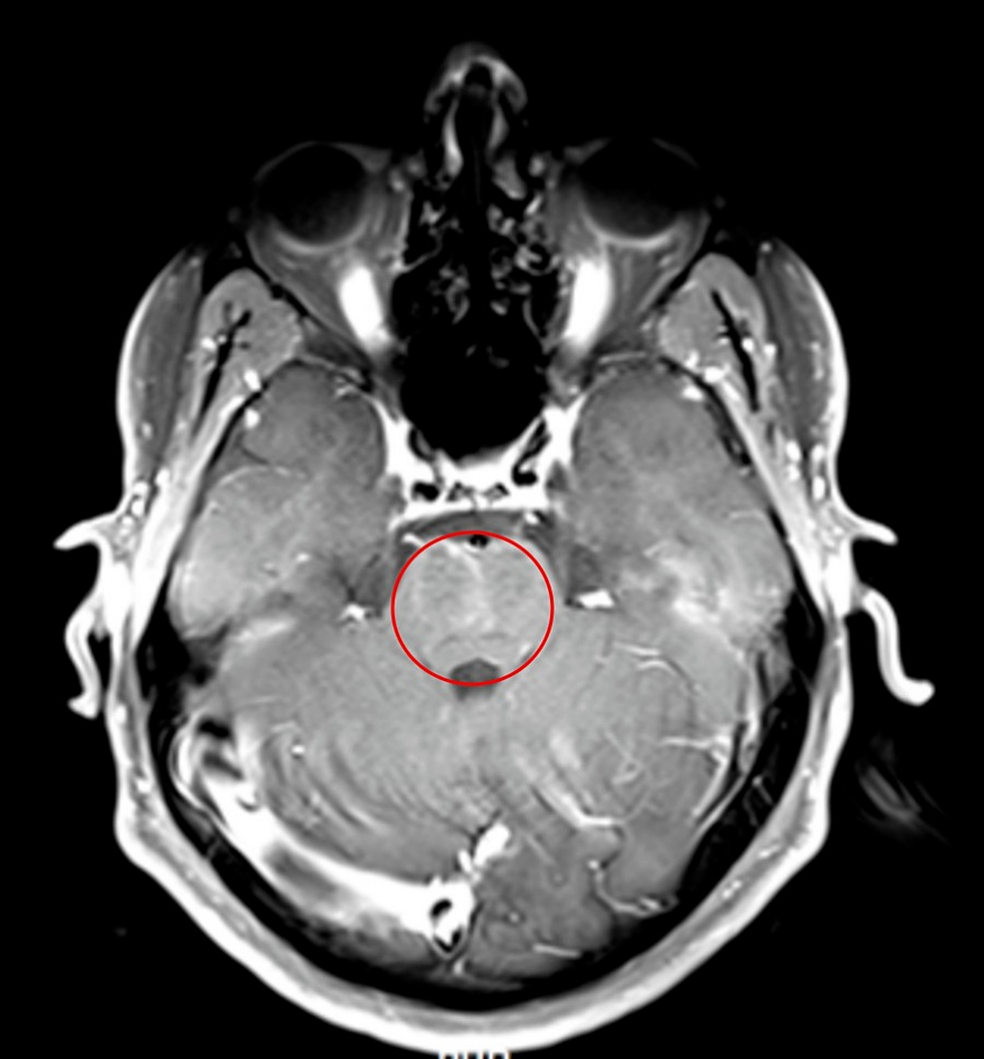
Axial MRI T1-weighted image with contrast sequence obtained post-treatment with pulse steroid showing signal improvement involving the pons (encircled). MRI: magnetic resonance imaging
